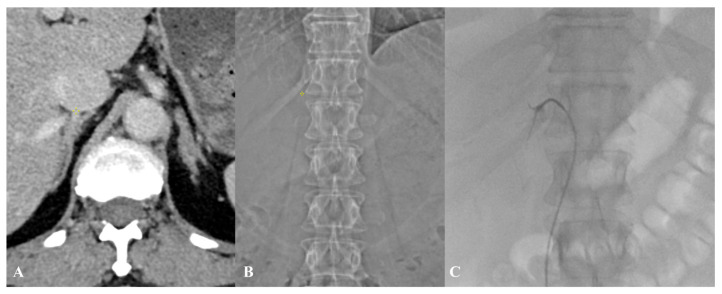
A 45-year-old male with confirmed primary aldosteronism. Using a 3D pointer (yellow symbol) on axial CECT images (A) and CT-scout (B), the RAV orifice was identified at the T12/L1 intervertebral level (C). During fluoroscopic AVS with free breathing, successful cannulation was achieved with a C1 catheter at the second quarter of T12 level, representing a cranial shift of three vertebral levels. The selectivity index achieved was 15.2.
